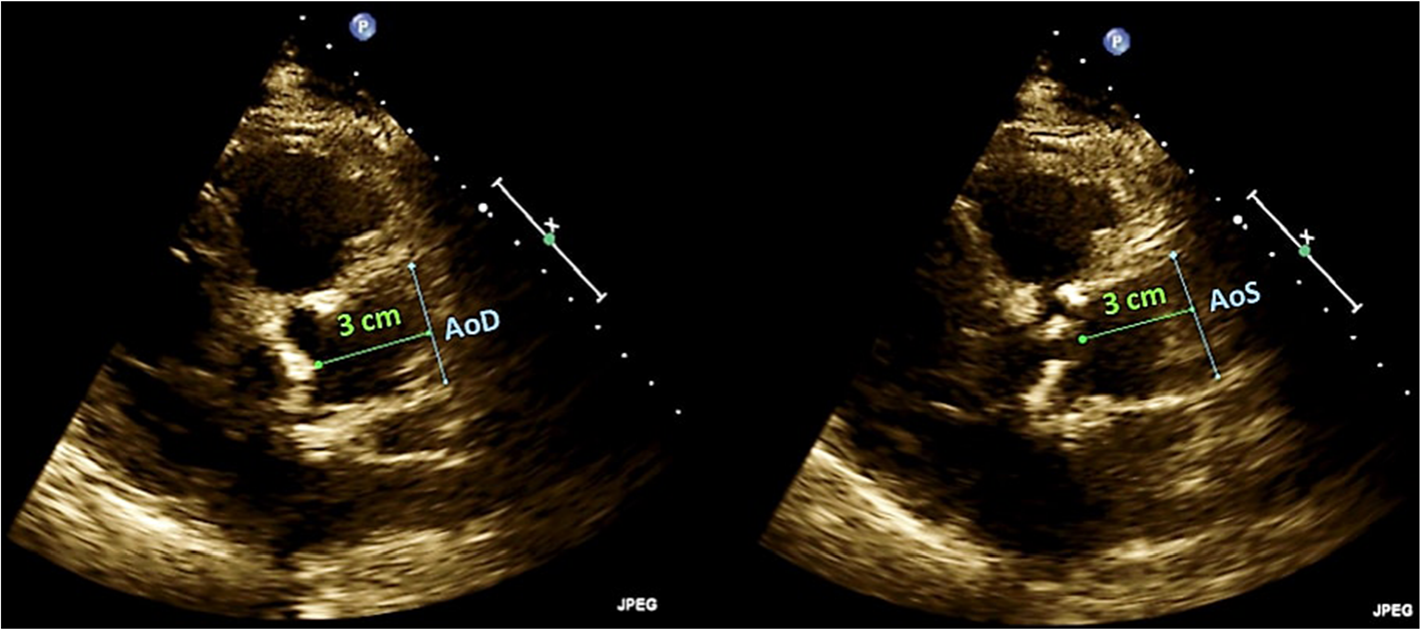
Ascending aortic diameter measurements. Ascending aortic diameters were measured three centimeters above the aortic valve at end-diastole (AoD) and end-systole (AoS) in the 2D parasternal view
